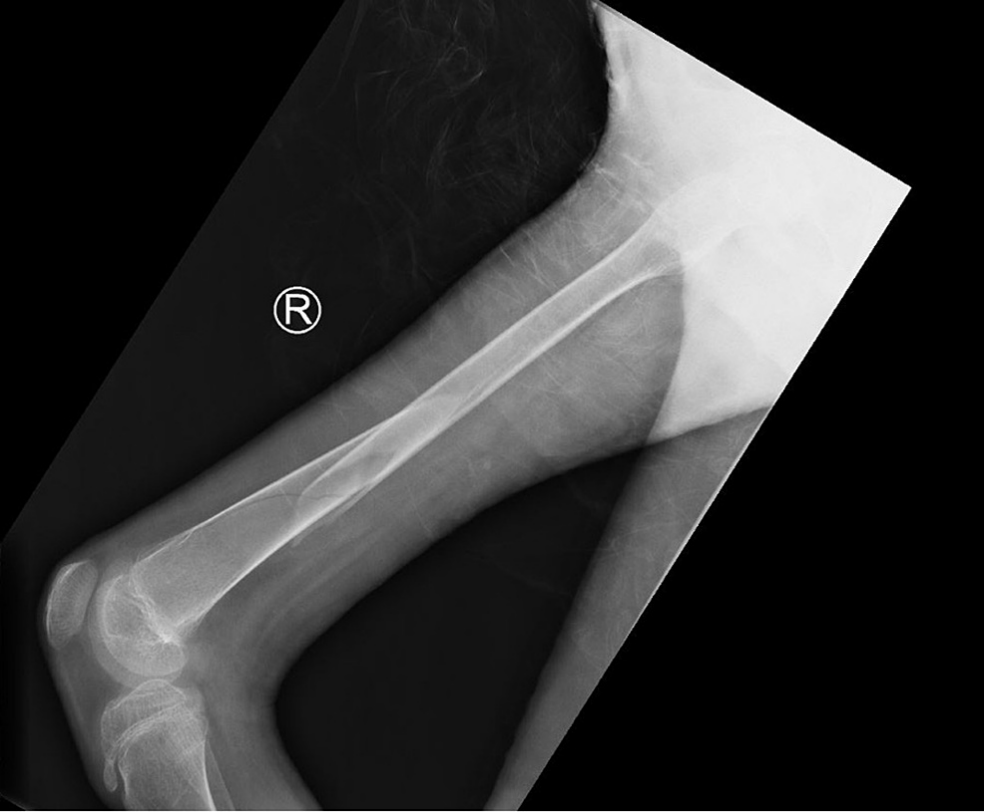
Lateral X-ray image taken on the day of the admission. Fracture of the femur and a crack in the distal diaphyseal region can be seen. However, the crack shown is not a fracture.
